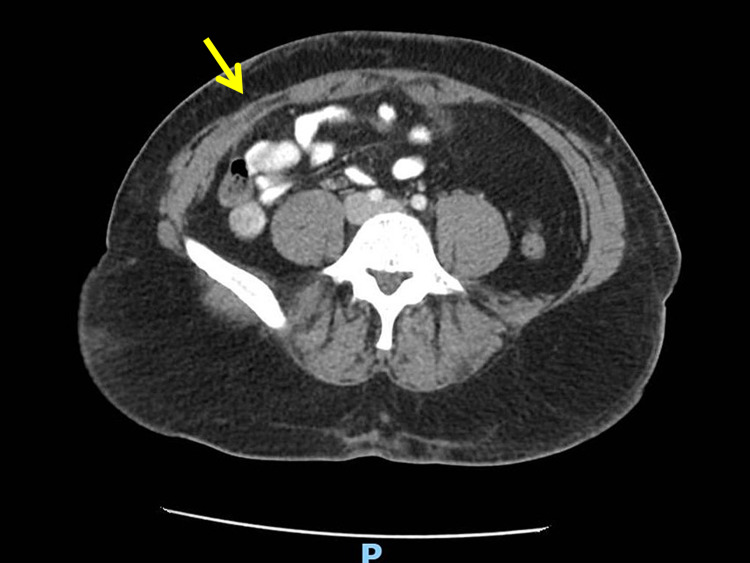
Postoperative CT of abdomen CT image of abdomen postoperatively revealing full resolution of fascial defect. No abdominal pathology is appreciated at this visit.
